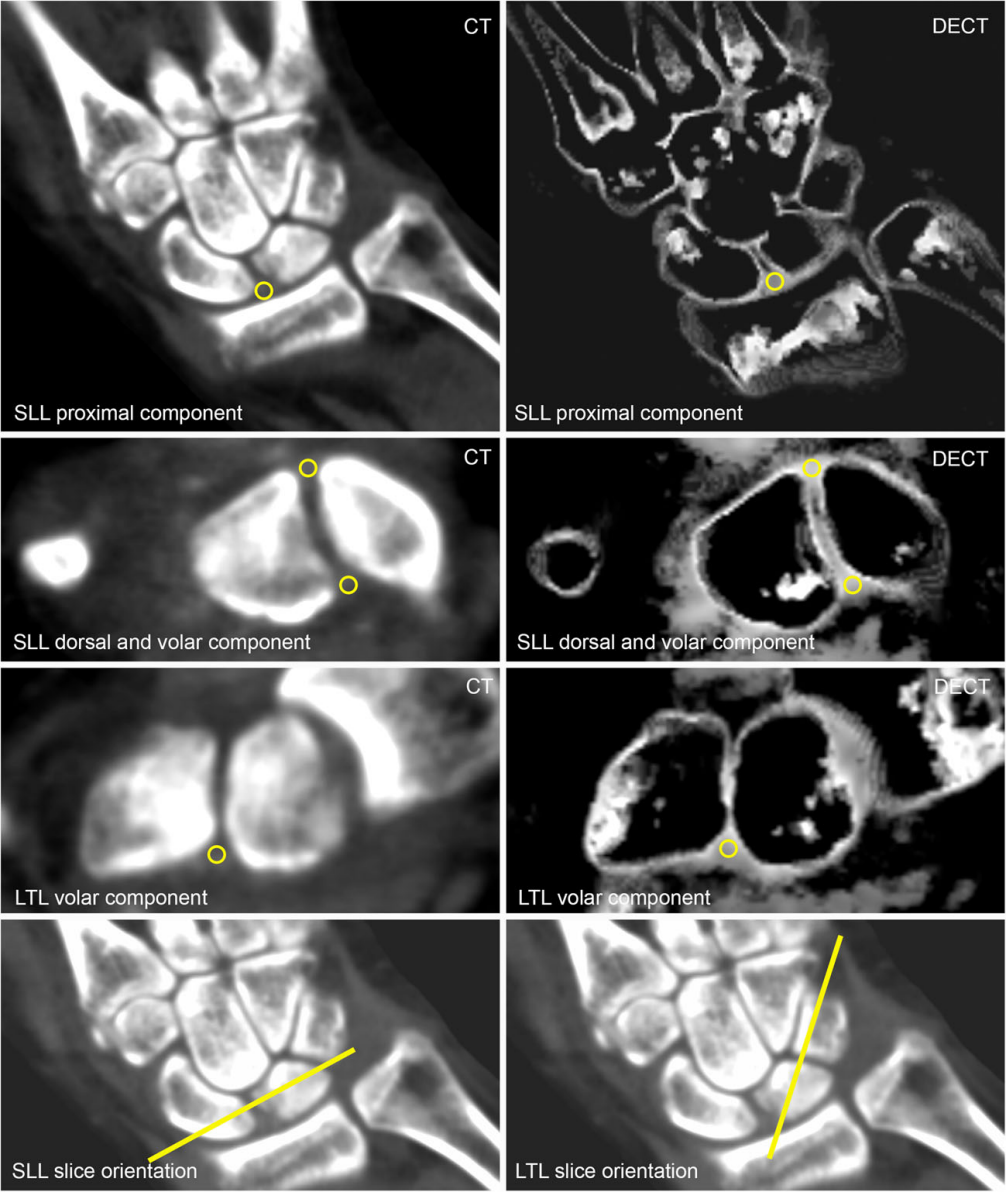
Measurement of collagen density. All images are from a 61-year-old female patient with gout. Left column: equivalent 120 kVp blended CT images. Right column: collagen maps. First row: example of ROI placement in proximal component of SL ligament. Second row: example of ROI placement in dorsal and volar component of SL ligament. Third row: example of ROI placement in volar component of LT ligament. Last row (both equivalent 120 kVp blended CT images): example of slice orientation for measurements
